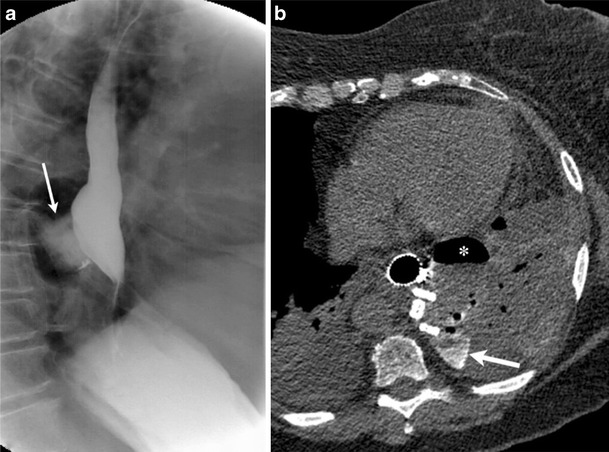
A 63-year-old male with oesophageal injury and resultant oesophageal-pleural fistula; post-Nissen fundoplication. Lateral image from contrast oesophagram using Omnipaque-350 (a) demonstrated extraluminal leak and subsequent mediastinal pooling (*arrow*) of orally administered contrast. Axial CT (b) demonstrates interval oesophageal stent placement. A complex left-sided paramediastinal fluid collection is seen containing both contrast (*arrow*) and air (*)
